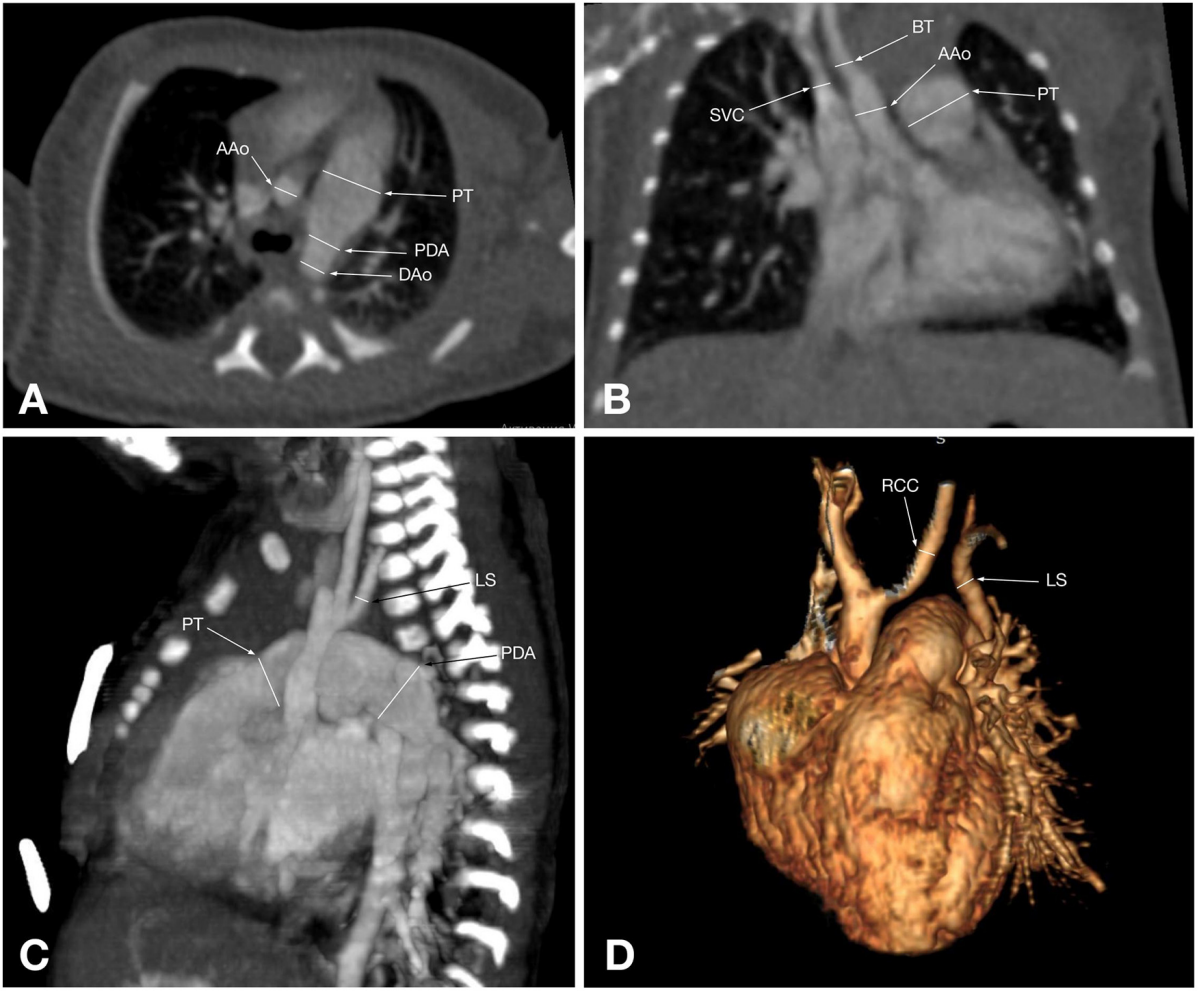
CT angiographic multiplanar images of a 55-day-old patient with type A IAA: (A) axial plane shows the branches of the aortic arch: brachiocephalic trunk (BT), left common carotid artery (LCC), and left subclavian artery (LS); (B) coronal plane shows that the ascending aorta branches into the brachiocephalic trunk (BT), which gives rise to the right common carotid artery. Also, the left common carotid artery (LCC) arising separately from the ascending aorta, the enlarged pulmonary trunk (PT), and its right branch are visualized; (C) Sagittal plane shows the pulmonary trunk (PT) continuing into the descending aorta via the patent ductus arteriosus (PDA); (D) 3D reconstruction shows an interrupted aortic arch after the left subclavian artery (LS) arises, corresponding to type A aortic arch interruption.
